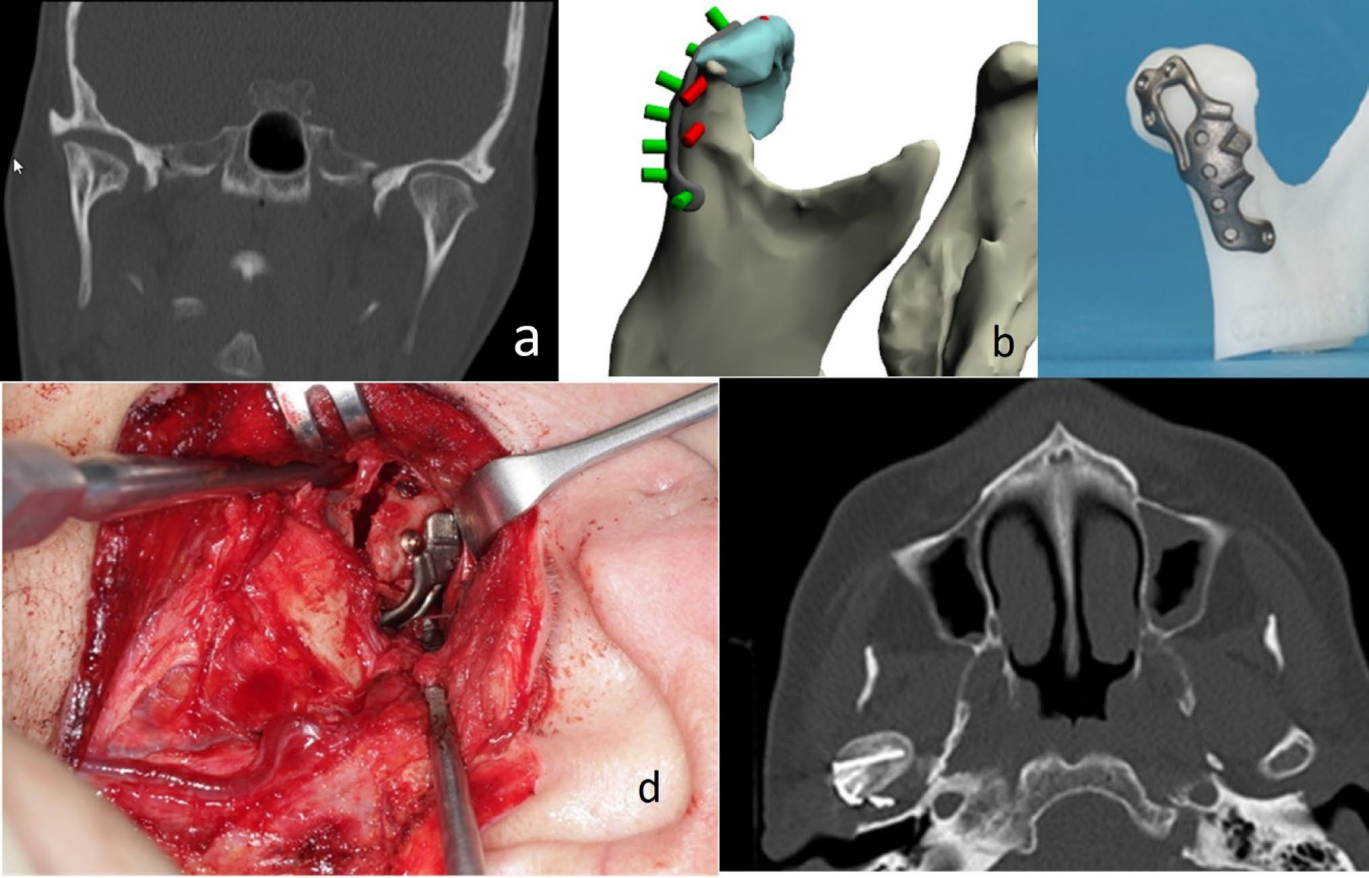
Case 3: Preoperative Panorex – deformity after conservative treatment and bone healing of the right condylar head following a displaced AO type-p CHF due to reduced vertical apposition of the medial fragment (a). Preoperative CT-Scan (coronal plane) – loss of vertical ramus height by caudal displacement of the medial fragment (b). VSP/CAD – reosteotomy and reduction of the medial condylar head segment (light blue); PSD (dark grey) fixed with micro screws (green) combined with LSFPSO via two screws (red) (c). STL-model with PSD (d). Intraoperatively – PSD in situ plus the heads of two screws for LSFPSO: one screw head next to the superior indicator notch, second screw head in more antero-superior position than planned (e). Postoperative CT-Scan (axial plane) (f)
